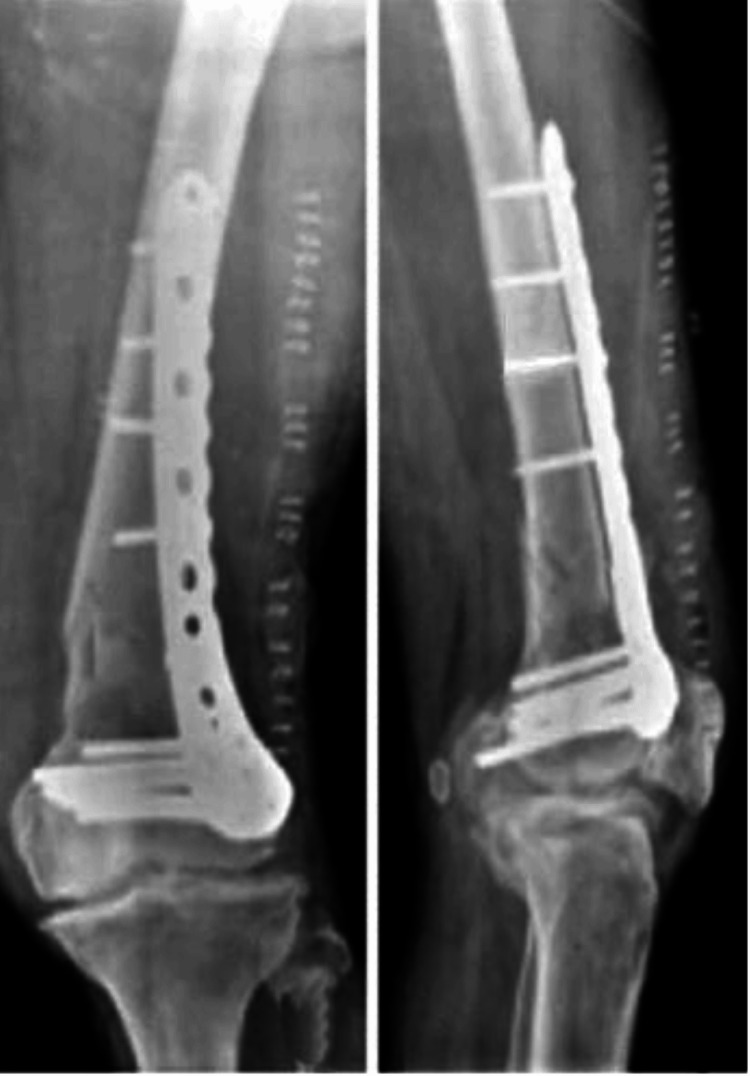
Case 2 immediate postoperative X-ray
